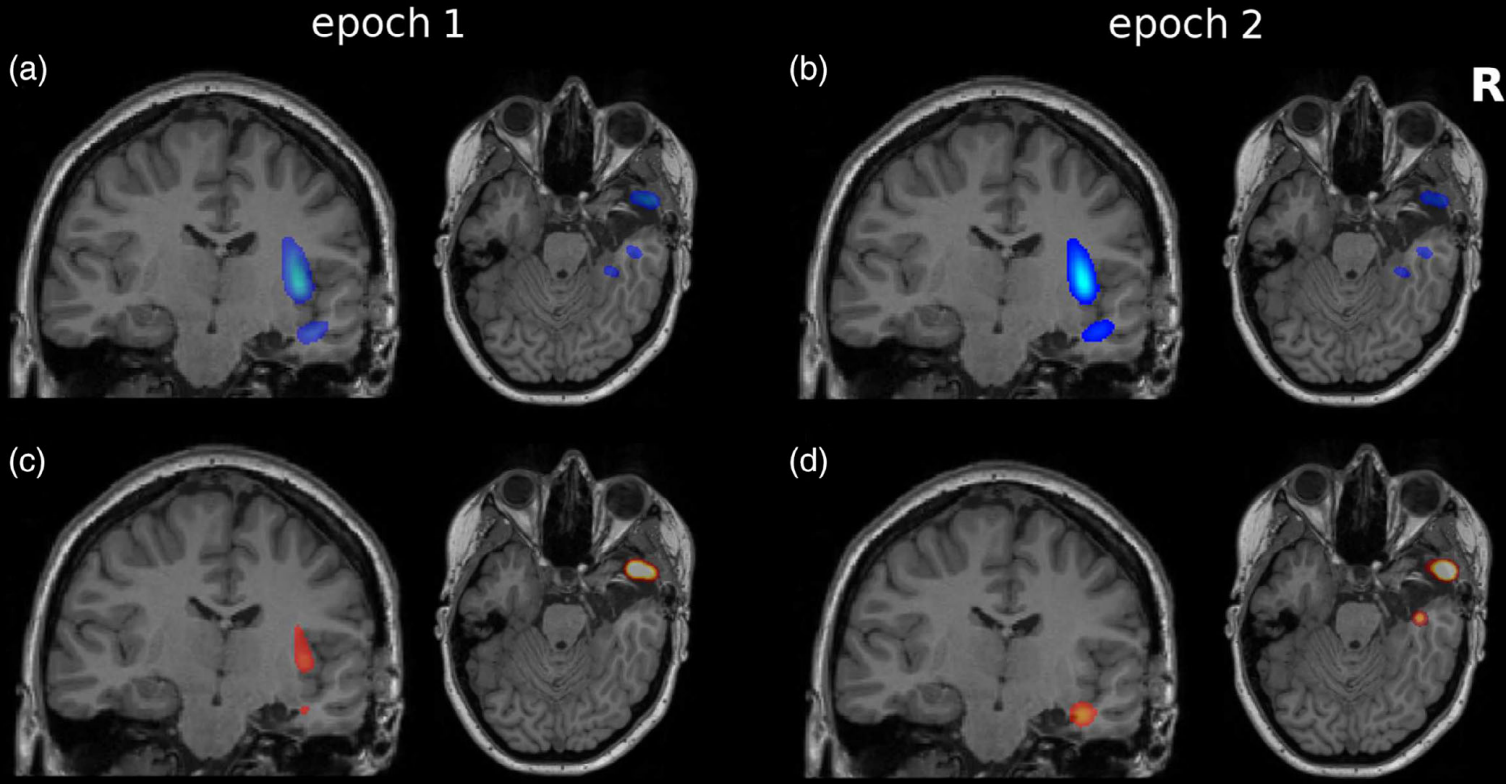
Transparent overlays of the node strengths of the linear (a,b) and nonlinear excess (c,d) interrelation matrices before seizure 1 (a,c) and seizure 2 (b,d) of Patient 13 over the postoperative T1‐weighted MRI, coronal, and axial slices. The extent of the resection is visible on the underlying MRI. For the corresponding matrices see Figure S4 [Color figure can be viewed at http://wileyonlinelibrary.com]
